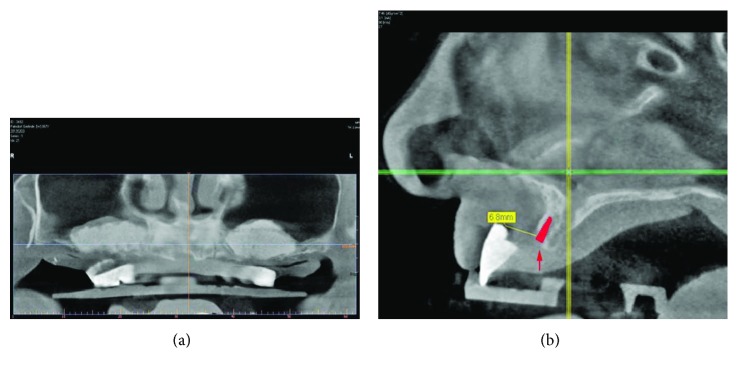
Implant planning. CBCT of the maxilla with DentDu-Brm *in situ* before implant placement: (a) sagittal section and (b) axial section.
